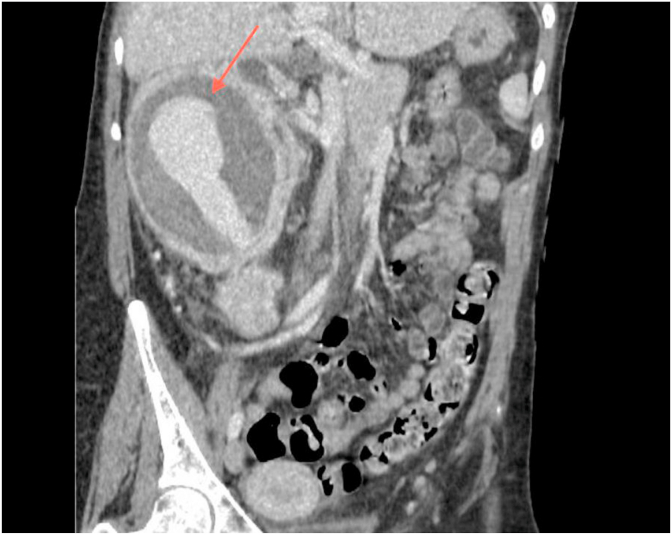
CT abdomen with contrast medium in coronal scan which in arterial phase shows active bleeding within the neoformation (red arrow). (For interpretation of the references to colour in this figure legend, the reader is referred to the Web version of this article.)
